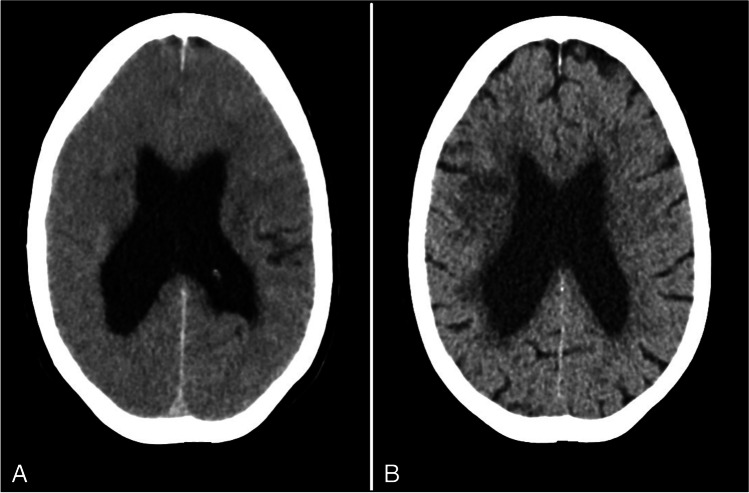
Case 1. Brain swelling on non-contrast CT performed directly after mechanical thrombectomy (A), which resolved on follow-up CT 4 days later (B)
